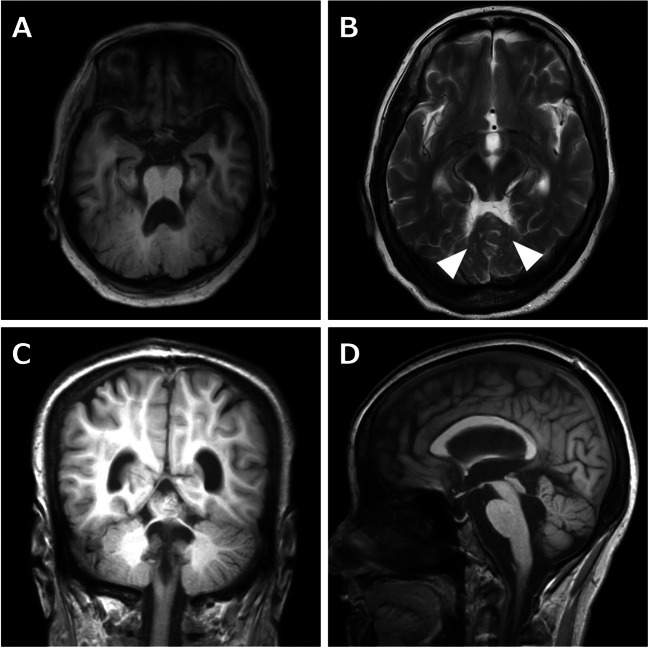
Magnetic resonance images (MRI): A Axial T1-weighted MRI, B Axial T2-weighted MRI, C Coronal T1-weighted MRI, and D Sagittal T1-weighted MRI. These images show elongated superior cerebellar peduncles without thickness or straight shape (A), multiple cerebellar cysts (B), splayed superior cerebellar peduncles (C), vermis hypoplasia, and rhomboid shaped fourth ventricle (D)
